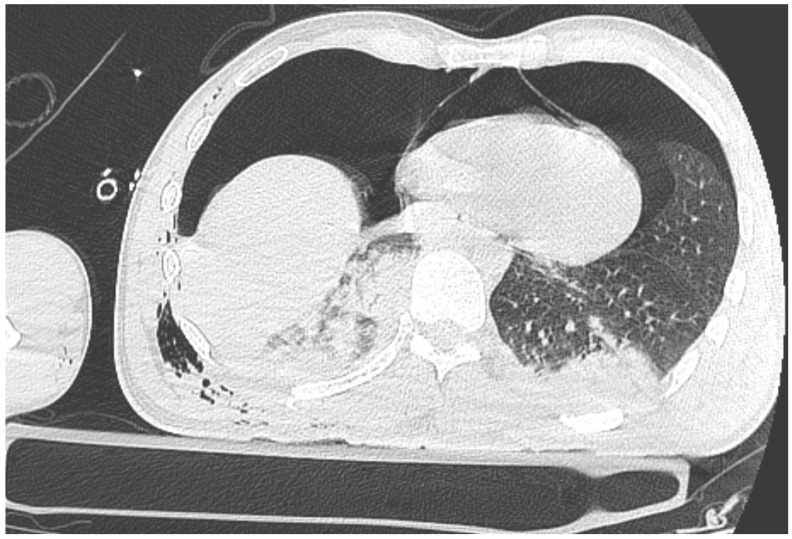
CT scan of pneumothorax and pneumocardium after a pneumopericardial decompression—scan at the level of the diaphragm.
